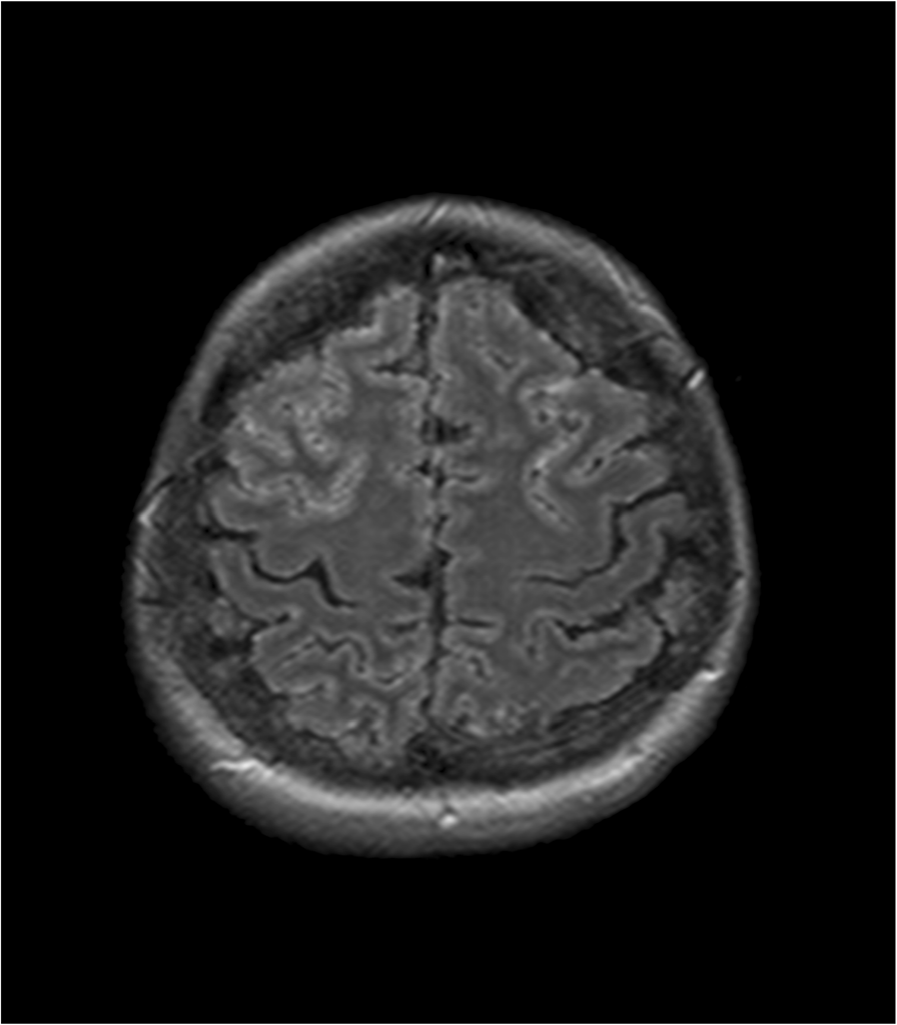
FLAIR sequence of MRI. The axial FLAIR sequence shows multiple small areas of signal hyperintensities in the grey matter and in the leptomeninges
